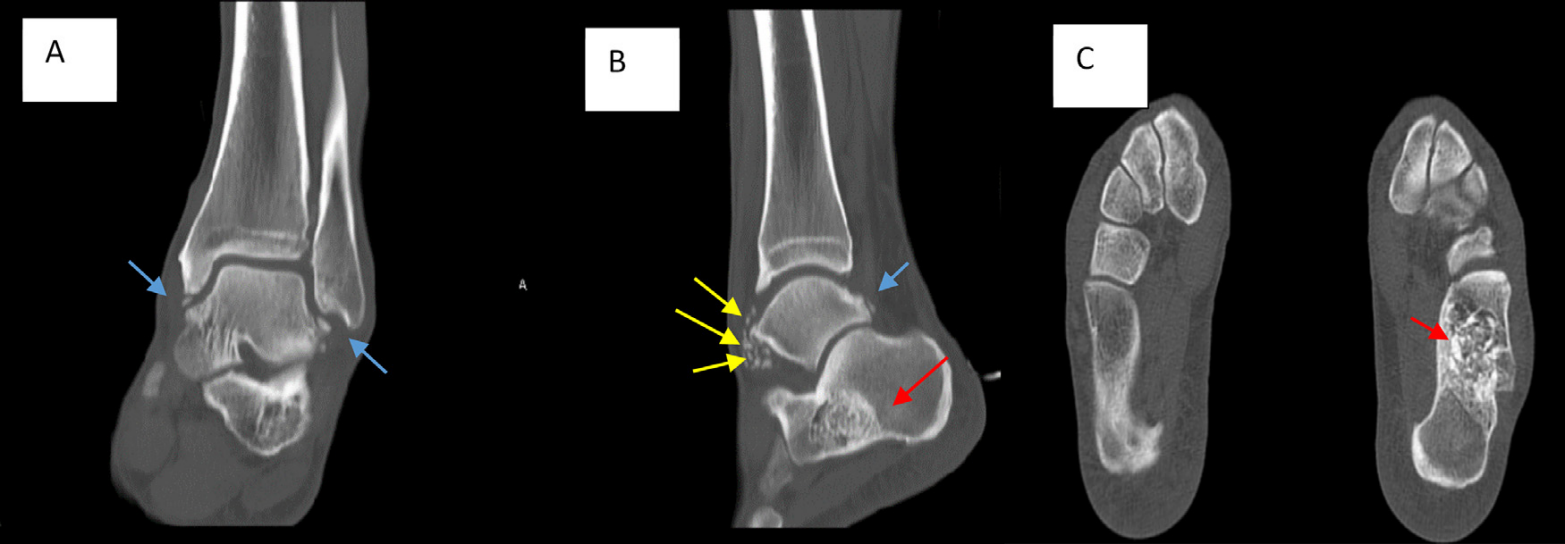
Computed tomography in A) Coronal View B) Sagittal View C) Axial View depicted that a lytic sclerotic lesion with a mixed density with a clear margin with a diameter of 57 mm is seen in the left calcaneus bone, inside which the appearance of the chondroid matrix is evident (Red arrow). Also, numerous uniform and ossified intra-articular loose bodies are seen in different parts of the left ankle, preferably in the anterior part of the talus and tibio-talar joint (Yellow arrows). Numerous osteophytes are seen in the distal tibia, talus, and fibula (Blue arrows).
